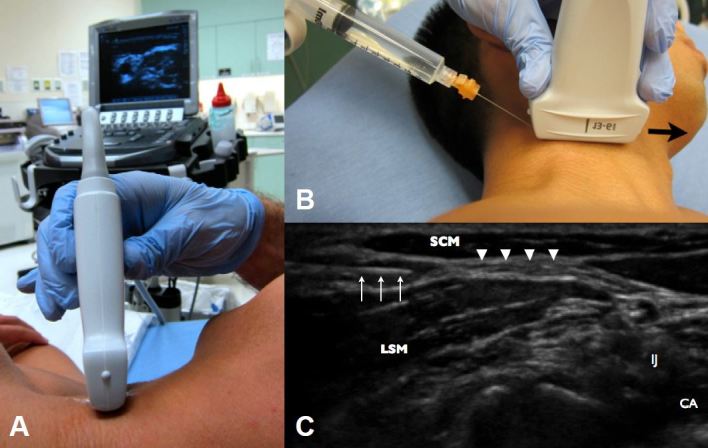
A*, Probe positioning for the in-plane approach in the lateral decubitus position. *B*, Needle injection and proper orientation of probe marker during superficial cervical plexus block. The arrow delineates the probe marker orientation. *C*, Ultrasound image of needle injection within the superficial cervical plexus. The arrows mark the needle. The sternocleidomastoid muscle (SCM) noted on the top right, the levator scapulae muscle on top left (LSM), the carotid artery (CA) and internal jugular vein (IJ) on the bottom right, and the injection site marked (arrowheads).
